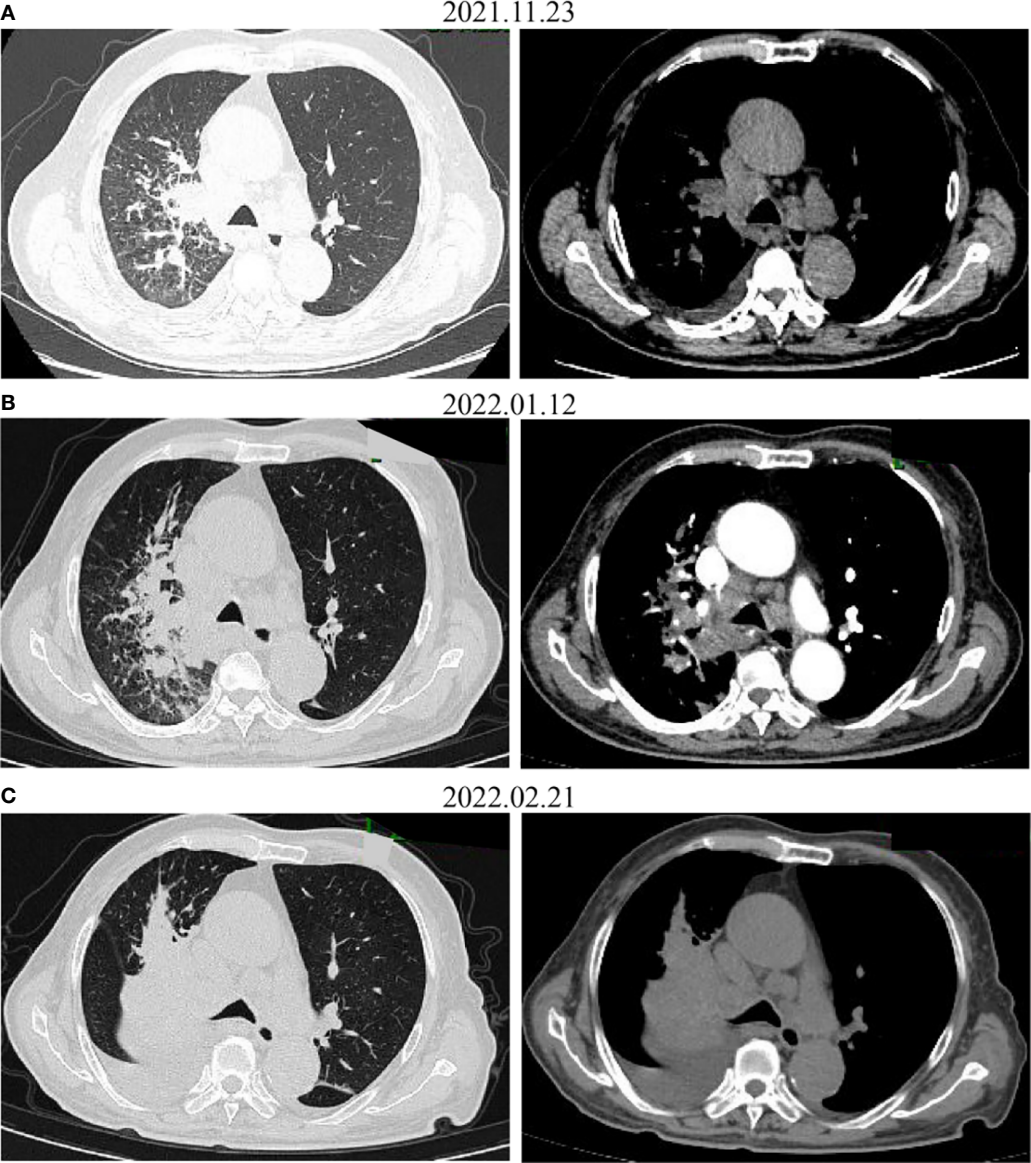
CT images before (A) and after administration of the first (B) and the second (C) dose with pembrolizumab. The tumor increased on CT.
